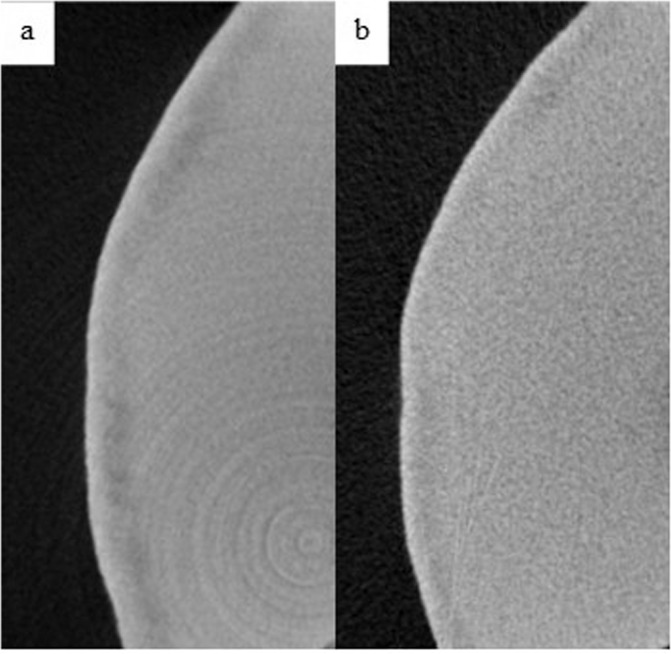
Remineralization of unetched enamel MIH lesions with 20% hydroxyapatite toothpaste. Representative µ-CT images of unetched enamel MIH lesions, before (a) and after (b) in-situ remineralization.
